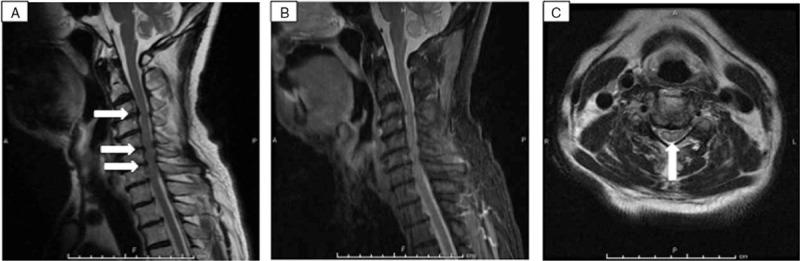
Magnetic resonance imaging (MRI) findings upon admission. A, Sagittal T2-weighted (3000/106.10 [TR/TE]) image shows degenerative changes at C3/4, C5/6, and C6/7 (arrow). B, Sagittal short T1 inversion recovery (STIR; 3500/16.91 [TR/TE]) image shows the same findings as in (A). C, Axial T2-weighted (3866.67/89.98 [TR/TE]) image shows hyperintense signal with involvement of grey matter and adjacent central white matter at C4/5 (arrow).
